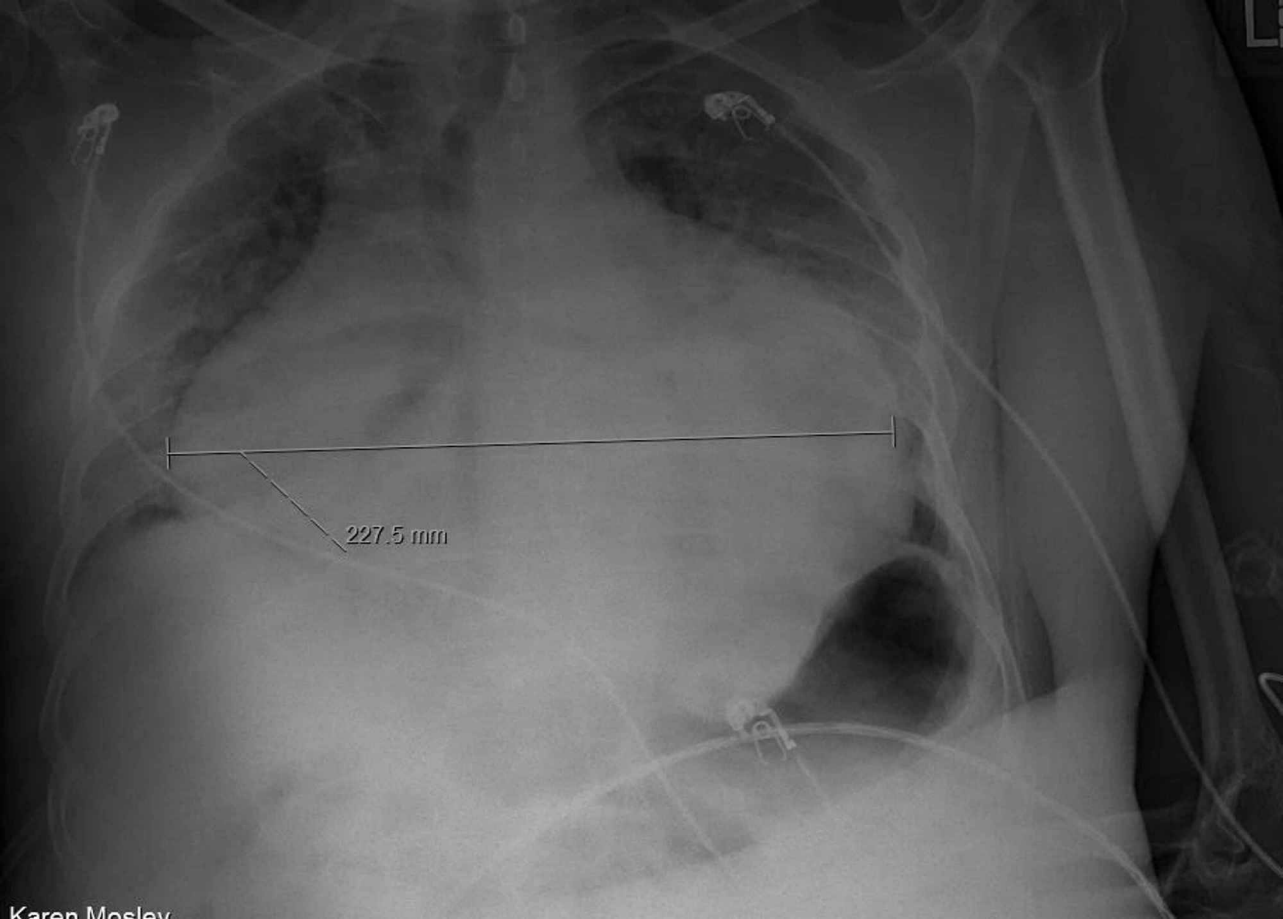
Chest X-ray shows cardiomegaly with diffuse bilateral infiltrates
